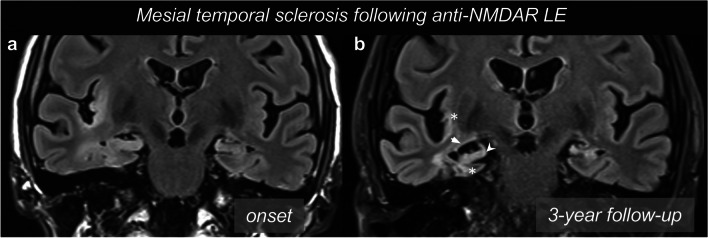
Medial temporal sclerosis as a sequela of limbic encephalitis. In this case of anti-NMDAR AE, T2-weighted FLAIR images (a) exhibited signal hyperintensity in the right MTL structures and insular cortex. The encephalitic insult evolved with atrophic changes over time. After three years, T2-weighted FLAIR images (b) showed medial temporal sclerosis, with volume loss of amygdala and hippocampus (arrowhead), as well as atrophy of the parahippocampus and insular cortex (asterisks), associated with an enlargement of the temporal horn of the lateral ventricle and choroidal fissure (arrow)
